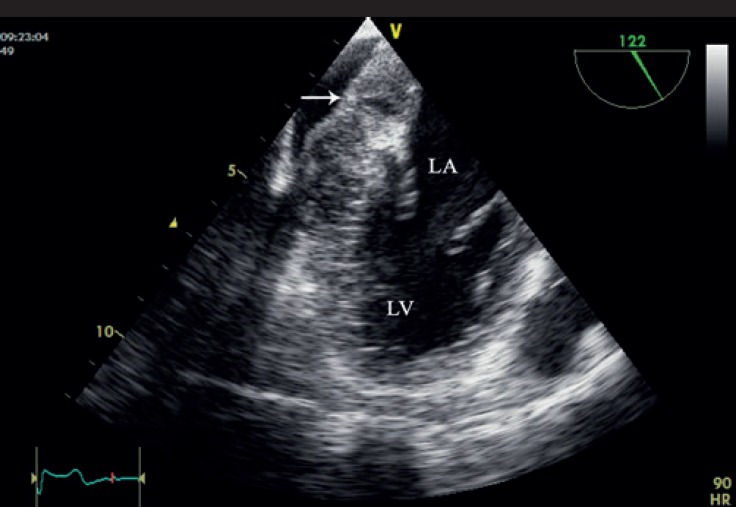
Transesophageal 2-dimensional echocardiography in the mid-esophageal view demonstrates a severely thickened pericardium (arrow).
